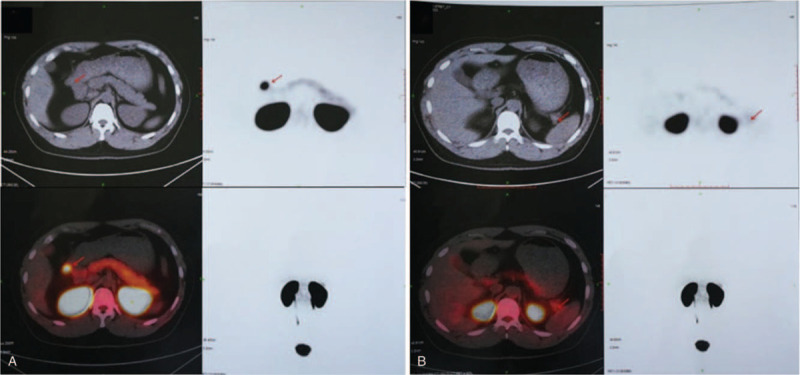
^68^Ga-Exendin-4 PET/CT showed intense uptake in the nodule on the right side of the gastric antrum, suggesting an insulinoma, no abnormal concentration of tracer was seen in the pancreas (A). The nodule in the tail of the pancreas showed no abnormal concentration of tracer (B).
